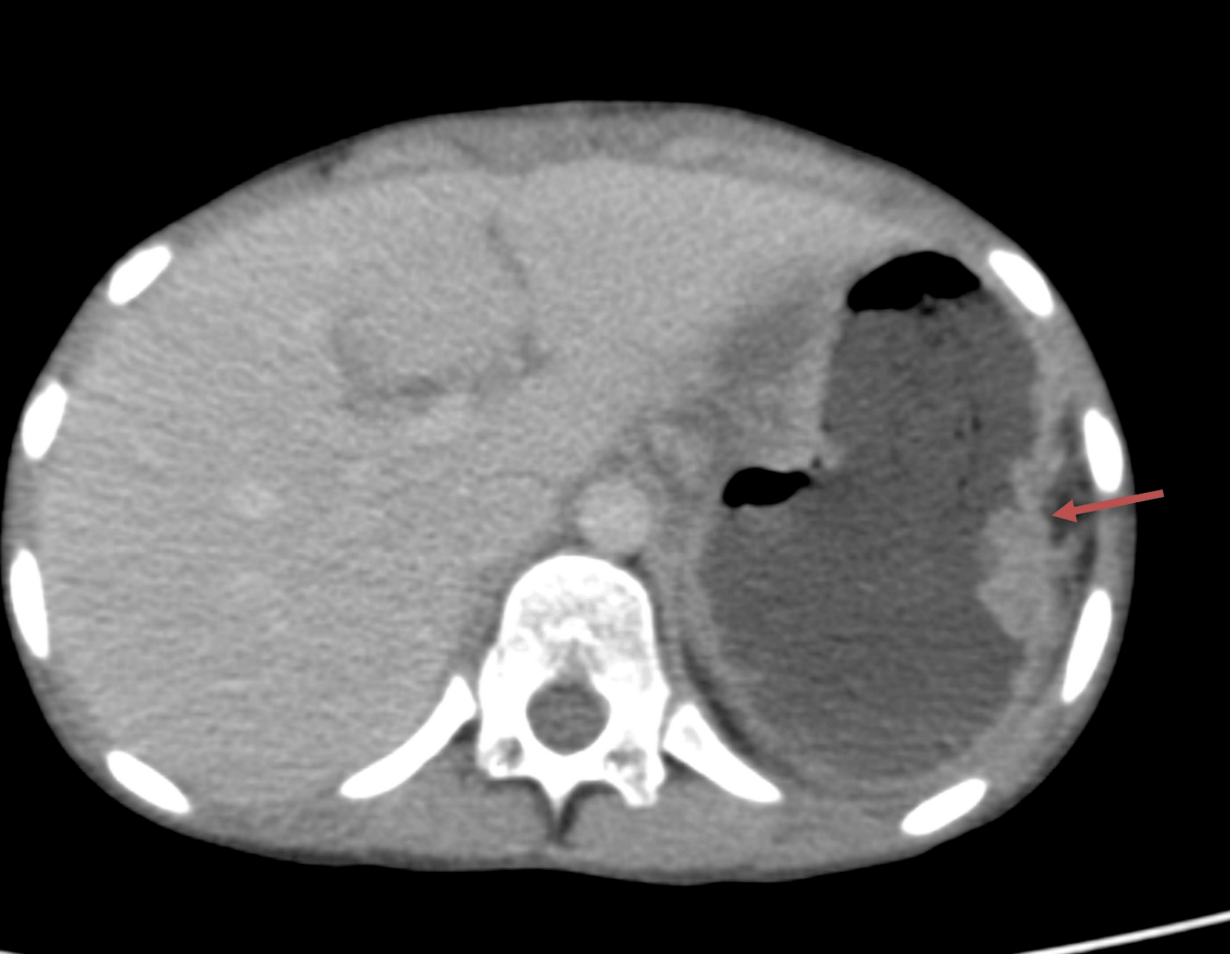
Postoperative computed tomography after six weeks showing mild thickening of the gastric wall
